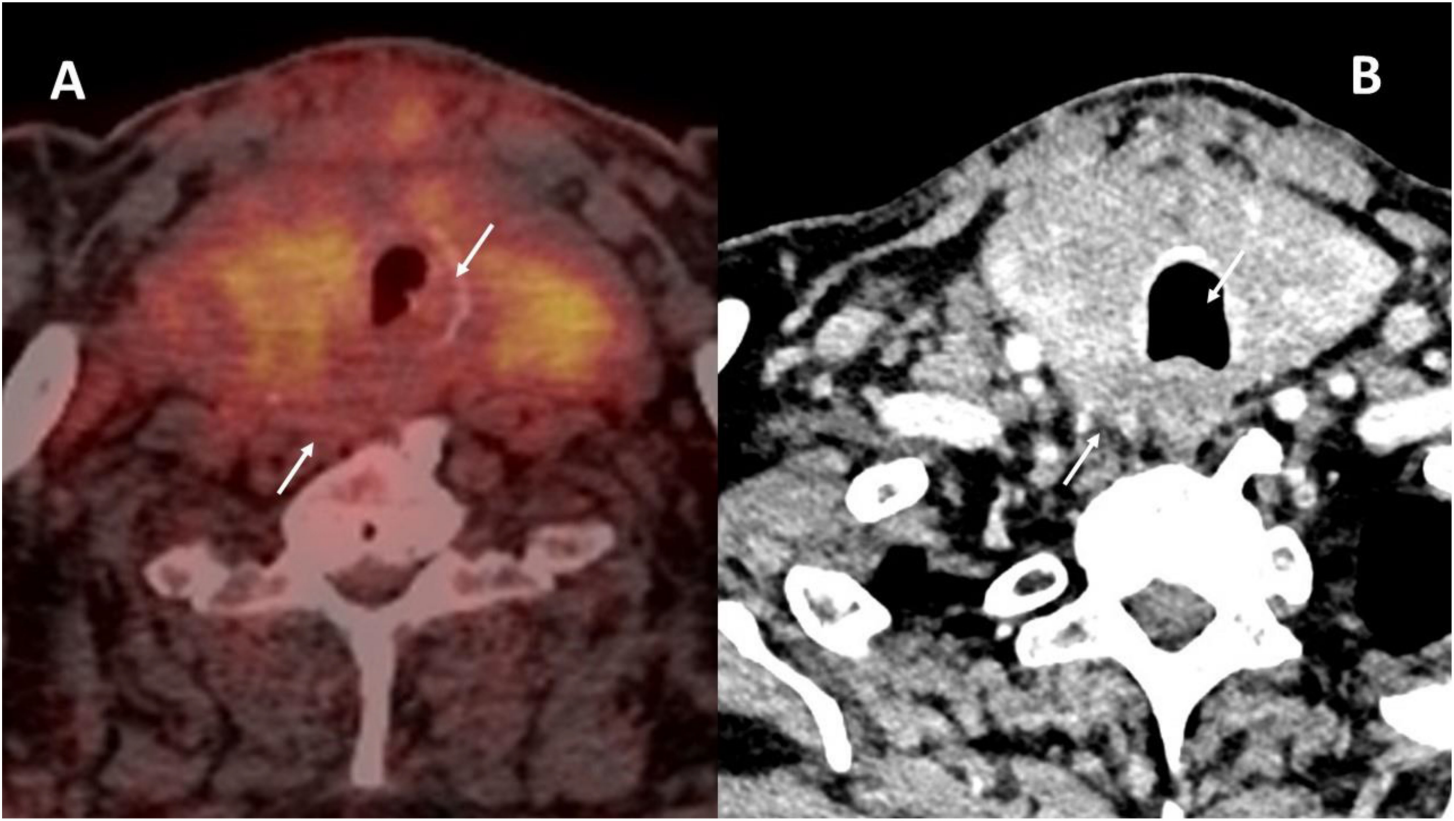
Tumor response in patient 2. Initial ^18^FDG-PET (A) as compared to the contrast-enhanced CT (B) after 4 months of cabozantinib and nivolumab. The initial tracheal invasion and involvement of the prevertebral muscles regressed with treatment (white arrows in both images).
